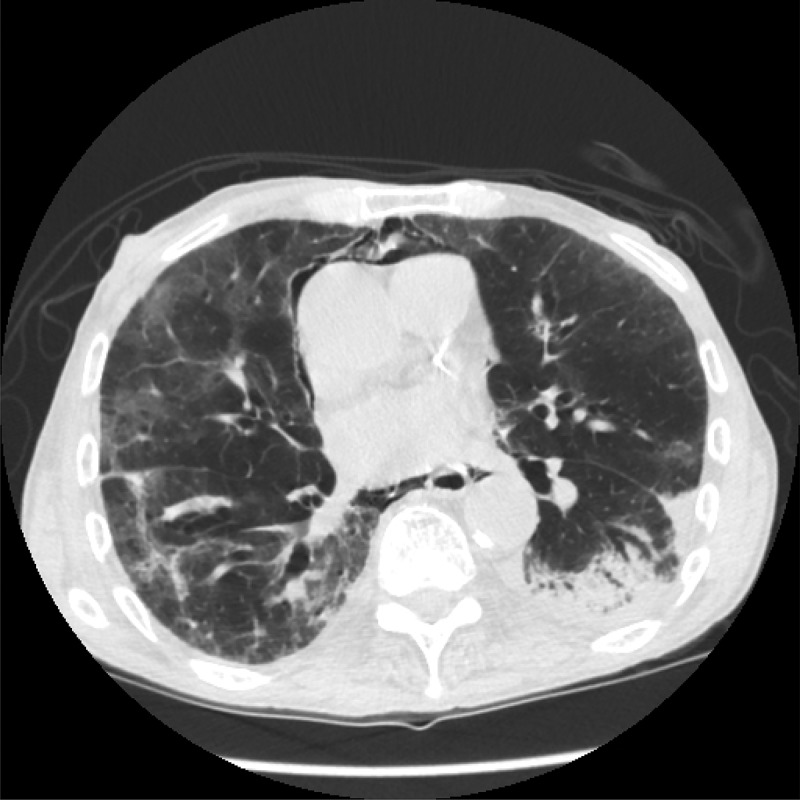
CT scan of chest showing improved opacity of lung parenchyma 2 weeks after discontinuation of daptomycin.
